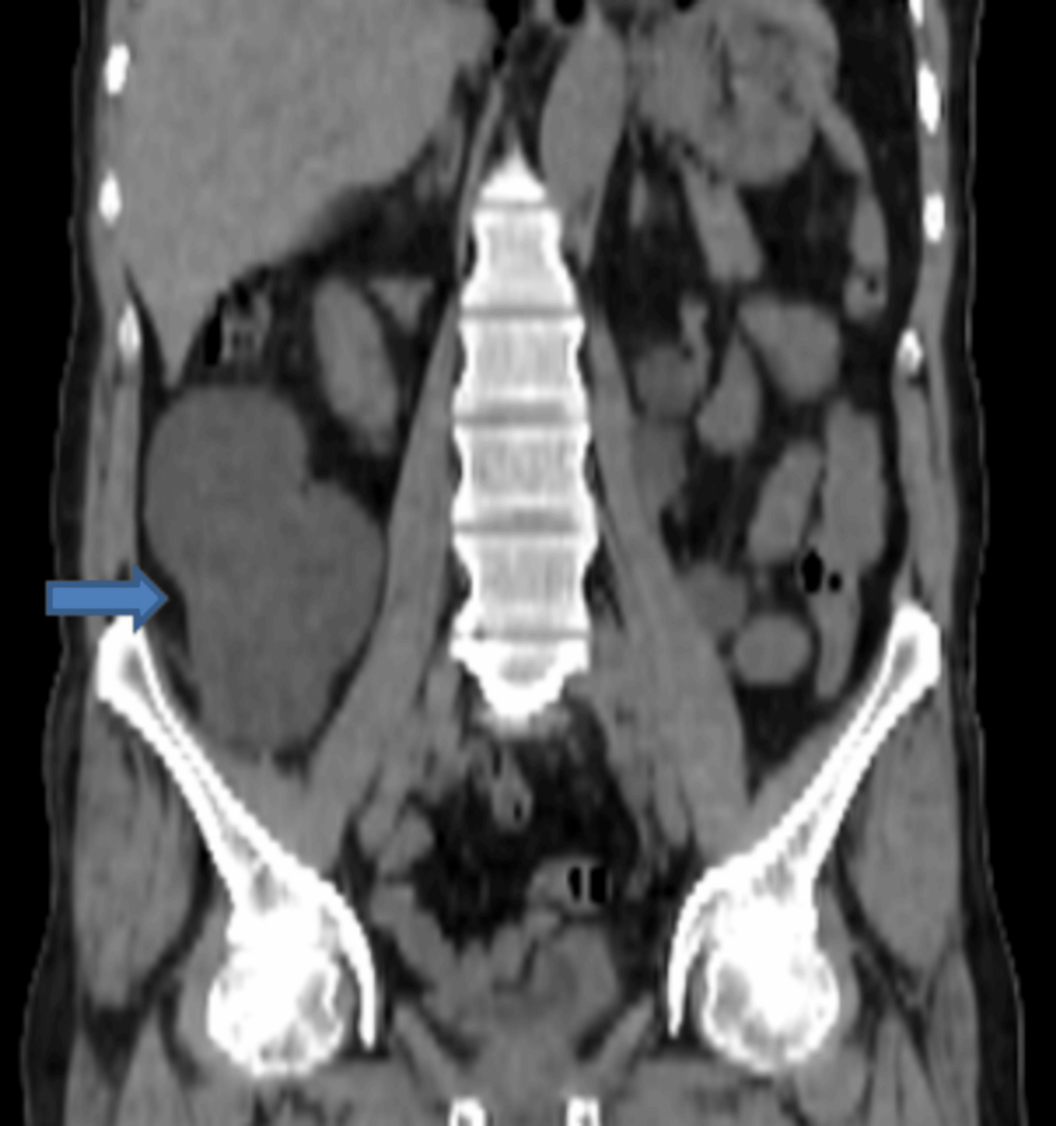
Non-contrast CT of the abdomen (coronal view) showing an encapsulated fluid collection in the right lumbar region (blue arrow). The collection appears to be inseparable from the right ureter with moderate dilatation of the right collecting system.
